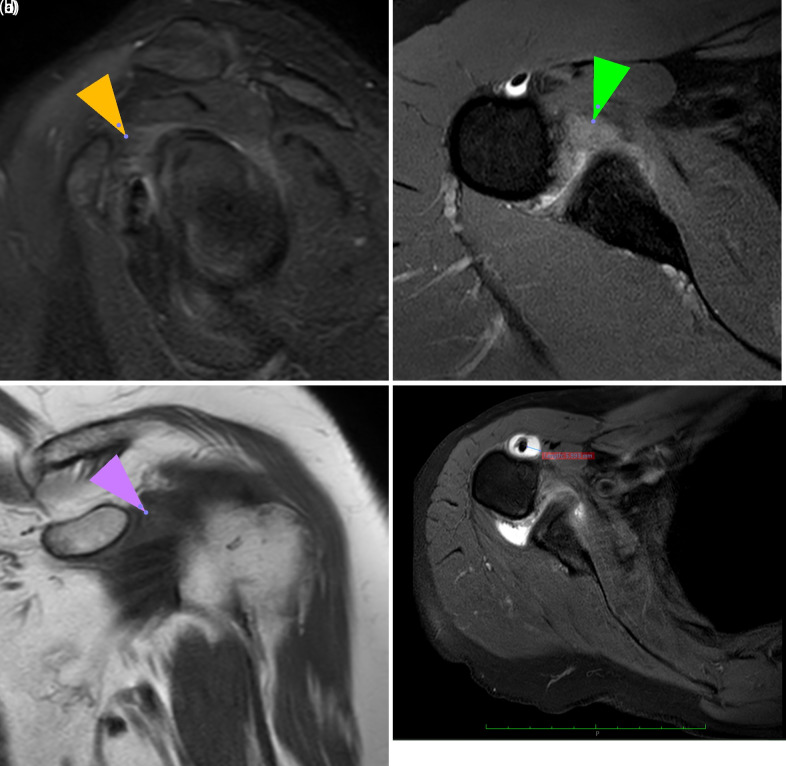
a. Edema observed in the sagittal T2-weighted fat-suppressed MR image at the rotator interval (yellow arrow). b. Edema observed in the axial T2-weighted fat-suppressed MR image at the axillary recess level of the joint capsule (green arrow). c. Fat tissue obliteration observed in the subcoracoid area on coronal T1-weighted MR image (purple arrow). d. Fluid surrounding the long head of the biceps tendon in the bicipital groove at the humeral neck (>2 mm) on axial T2-weighted fat-suppressed MR image.
